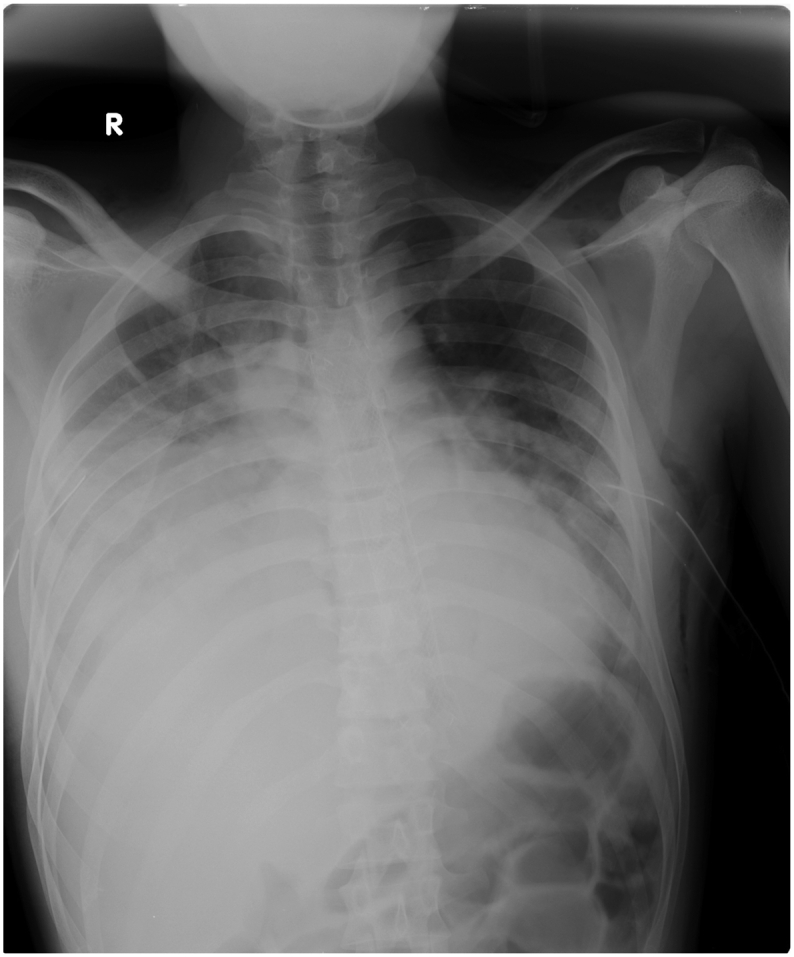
Chest radiograph showing right-sided pleural effusion and pneumomediastinum.
